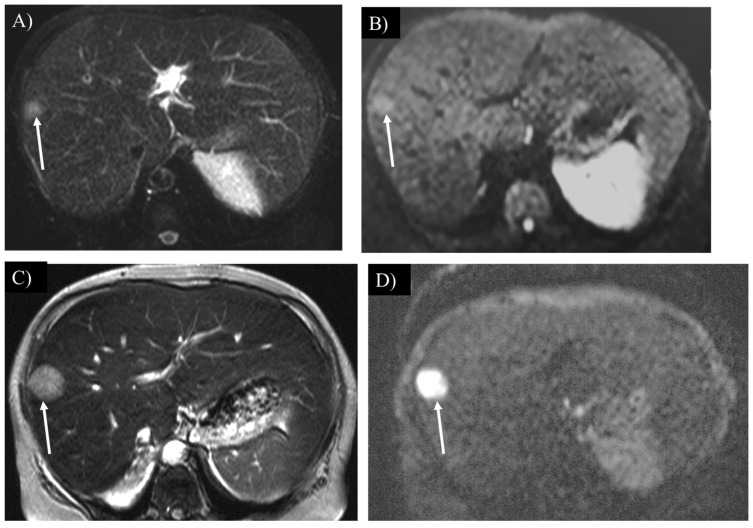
A liver tumor shows high intensity on T2-weighted (A) and diffusion-weighted imaging (B) in a 55-year-old woman with glycogen storage disease type Ia (arrow). The tumor grew rapidly over 1 year (C), (arrow). The tumor shows marked hyperintensity on diffusion-weighted imaging (D), (arrow), which is pathologically proven to be hepatocellular carcinoma.
